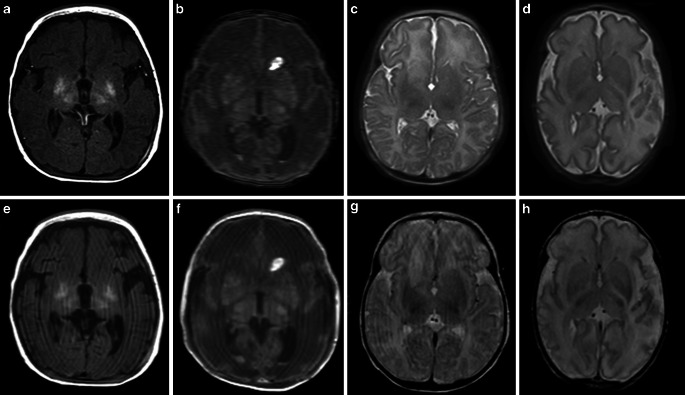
Selection of pathological cases. The *upper row* shows conventional MR contrasts (a–d). The *bottom row* shows SyMRI-generated MR contrasts (e–h). a, e Hypoxic ischemic encephalopathy in a term-born infant (39 + 4 weeks) (T1-weighted contrast). b, f Former preterm neonate (34 + 6 weeks) with multifocal intracranial hemorrhage (T1-weighted contrast). c, g Term-born infant (41 + 5 weeks) with expired infarction in the right caudate nucleus (T2-weighted contrast). d, h Former preterm neonate (32 + 4 weeks) with infarction of the left hemisphere (T2-weighted contrast)
